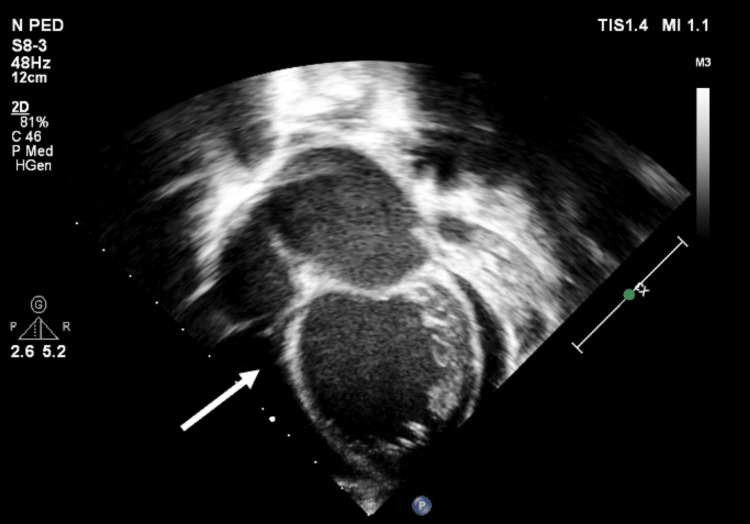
Follow-up echocardiography after six months showing minimal pericardial effusion (apical four chamber view)
